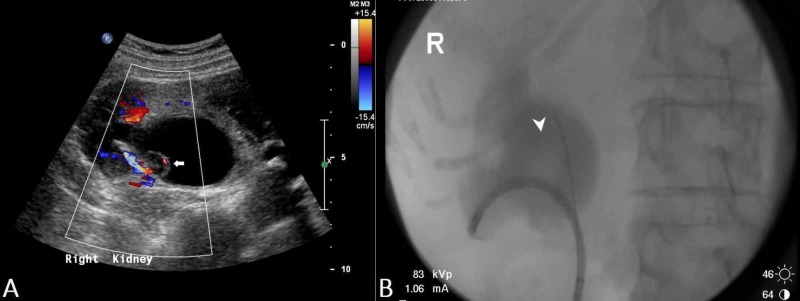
Renal transitional cell carcinoma (US and RPUG) (A) Ultrasound pelvis demonstrates a diffusely thickened proximal right ureter with a papillary lesion (arrow) seen within the right renal pelvis, and resultant right hydronephrosis. (B) This corresponds to the retrograde pyelo-ureterogram (RPUG) findings (arrow head) which is suggestive of an obstructing right upper urinary tract transitional cell carcinoma.
